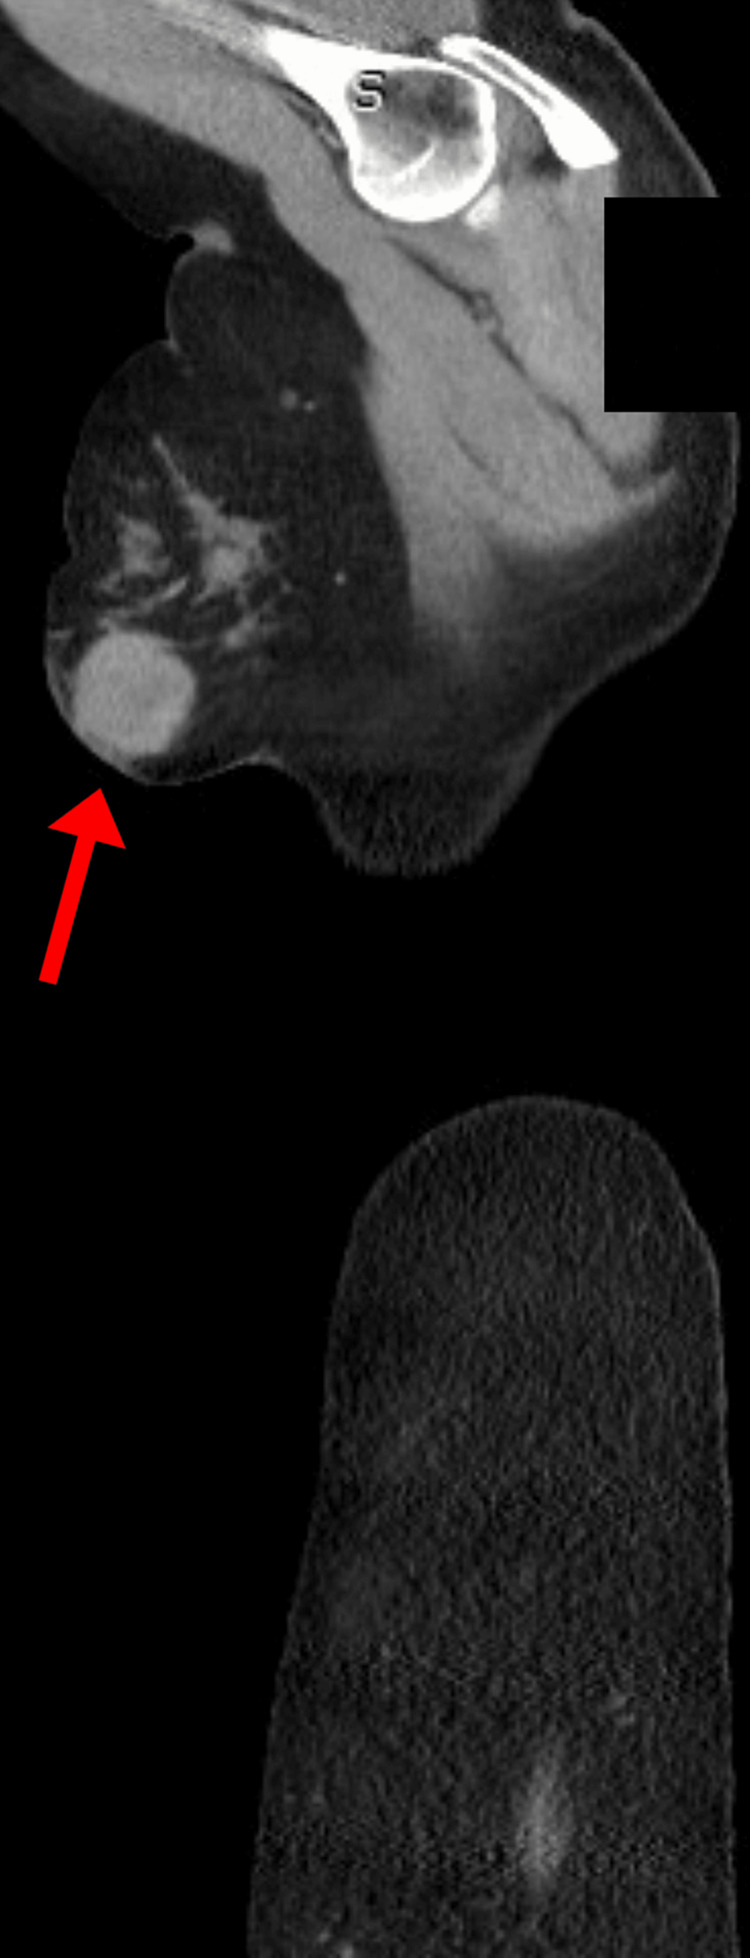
CT scan showing a 4.2-cm right breast mass (sagittal view)
